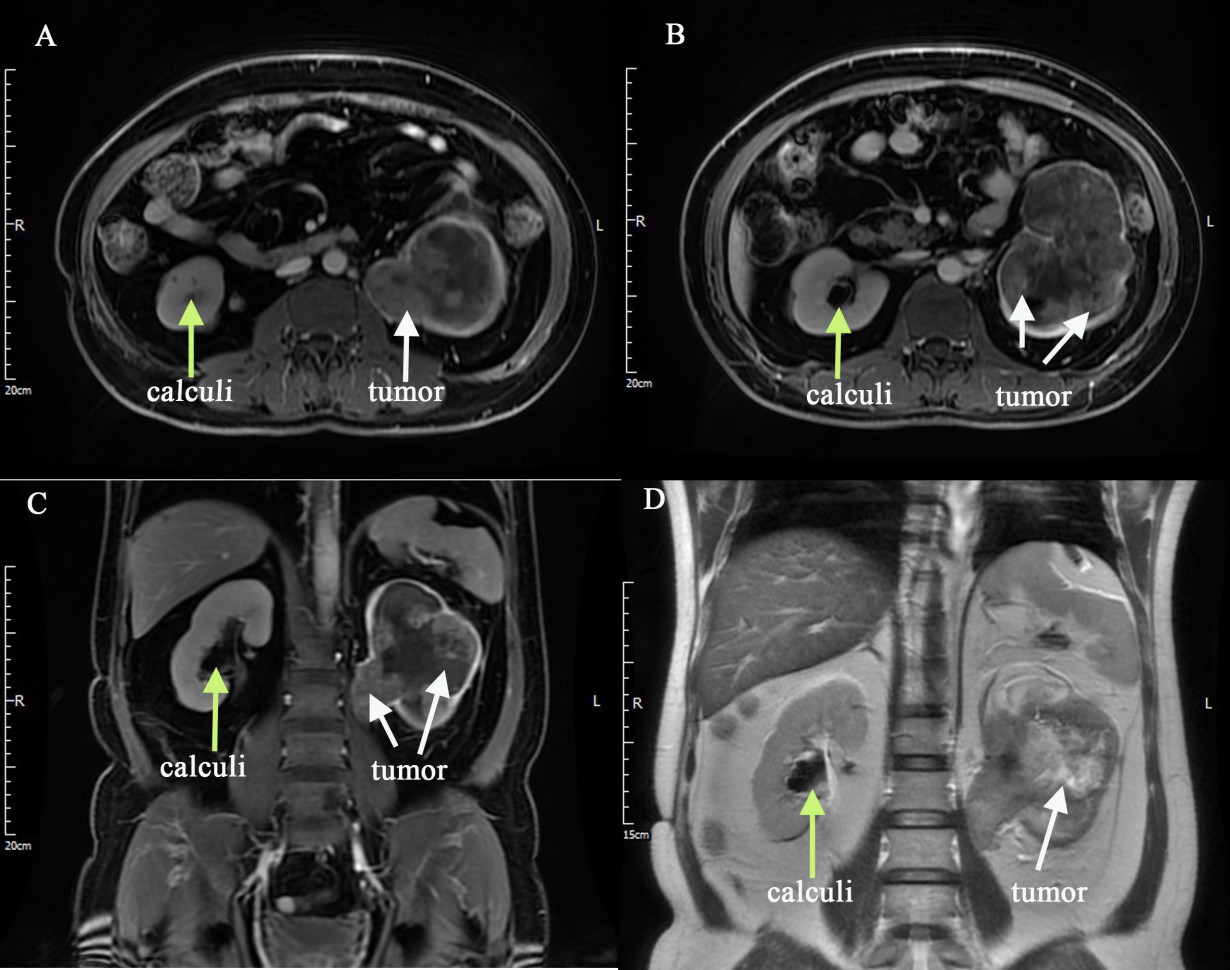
Contrast-enhanced MRI scan was performed (A–D), and it showed an enlarged left kidney, and a soft tissue mass was seen in the renal pelvis, calyces and upper ureter, and with bilateral renal calculi. (The tumor was showed by white arrows, and the calculi was showed by yellow arrows).
